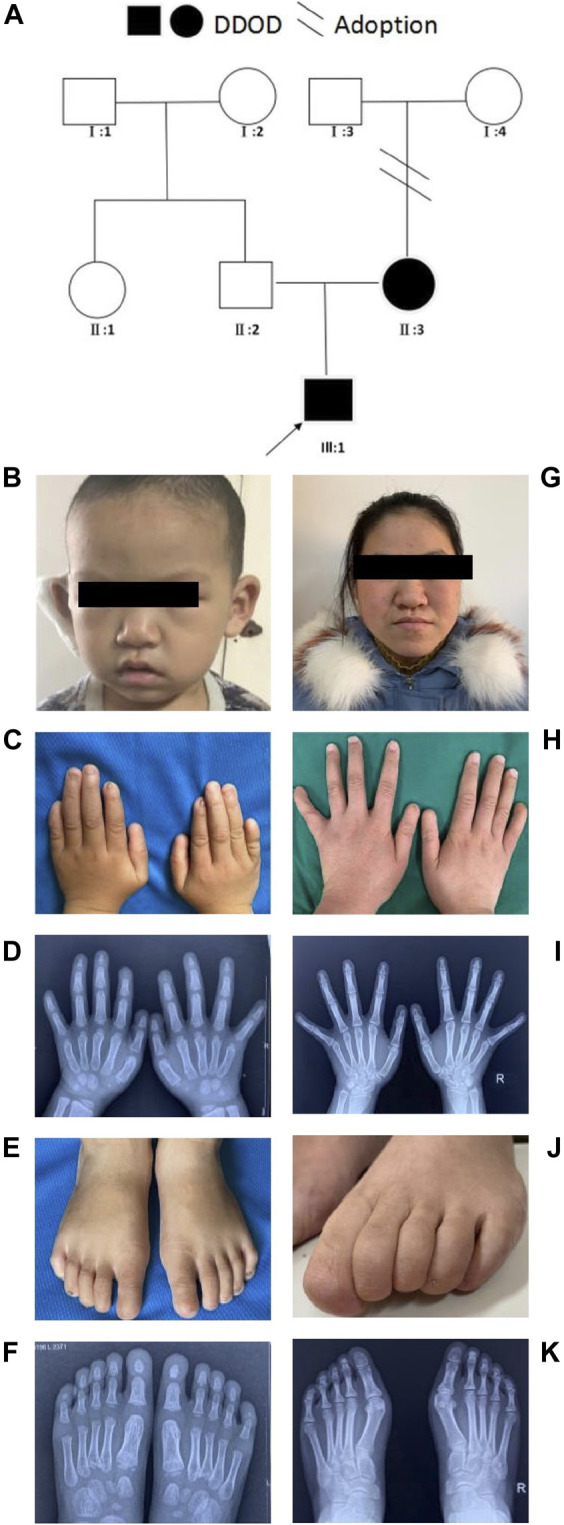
Clinical findings of family 1. (A) Family tree. (B–F) Proband and onychodystrophy. In fingers (C,D), absence of first- and fifth-digits fingernails, index fingers aplasia, finger-like thumbs, and shorter fifth fingers. In toes (E,F), absent toenails on the first and second digits, and hypoplasia toenails on the third to fifth digits of right foot; Left foot, absent toenails on the digits 1–3, rudimentary digits 4–5. Hand X-ray: distal phalanges absence of the index finger; hypoplasia of the distal phalanx of feet’s fifth digits. (G–K) Phenotypes of onychodystrophy of the mother are similar to the proband.
